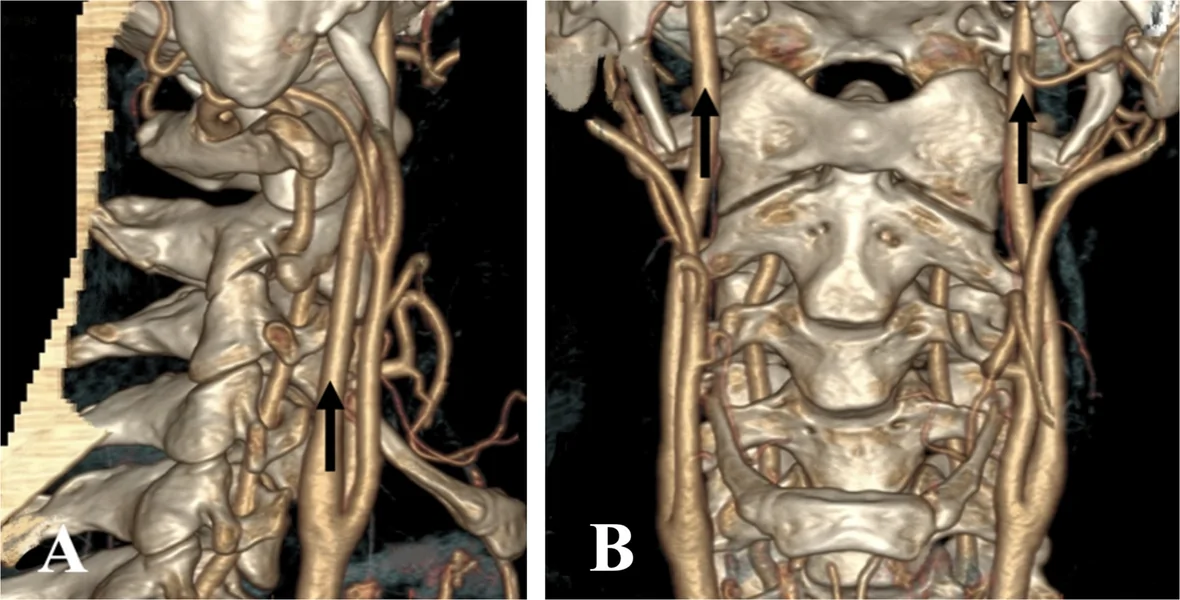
CT angiography showing the straight pattern (black arrow) of the cervical portion of the internal carotid artery. A. Sagittal view, B. Frontal view
